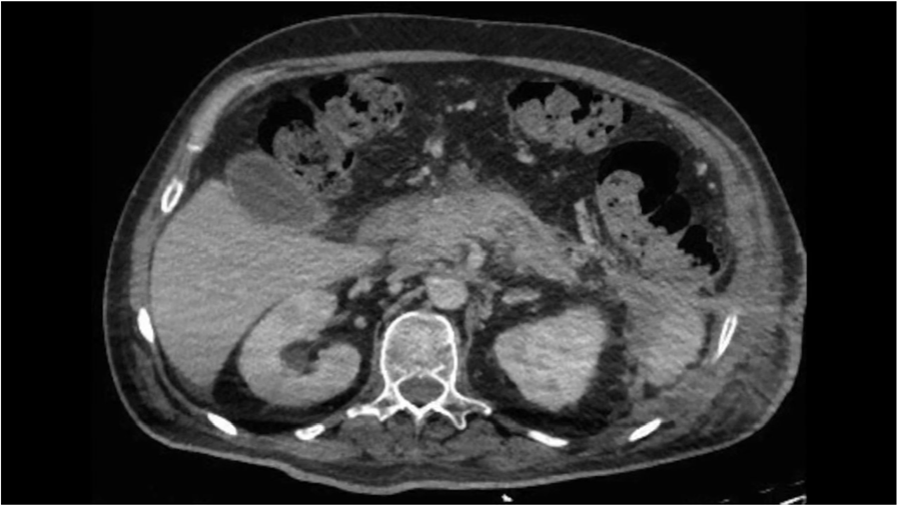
CT scan after SEMS removal showing a complete resolution of the collection
